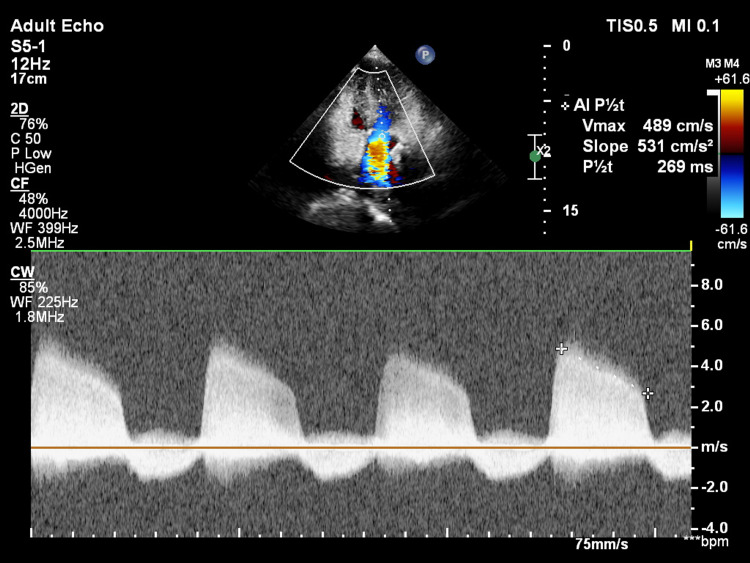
Continuous wave Doppler across the aortic valve showing an aortic regurgitation pressure half-time of 269 ms. AI P1/2t: aortic insufficiency (regurgitation) pressure half-time; Vmax: maximum velocity
